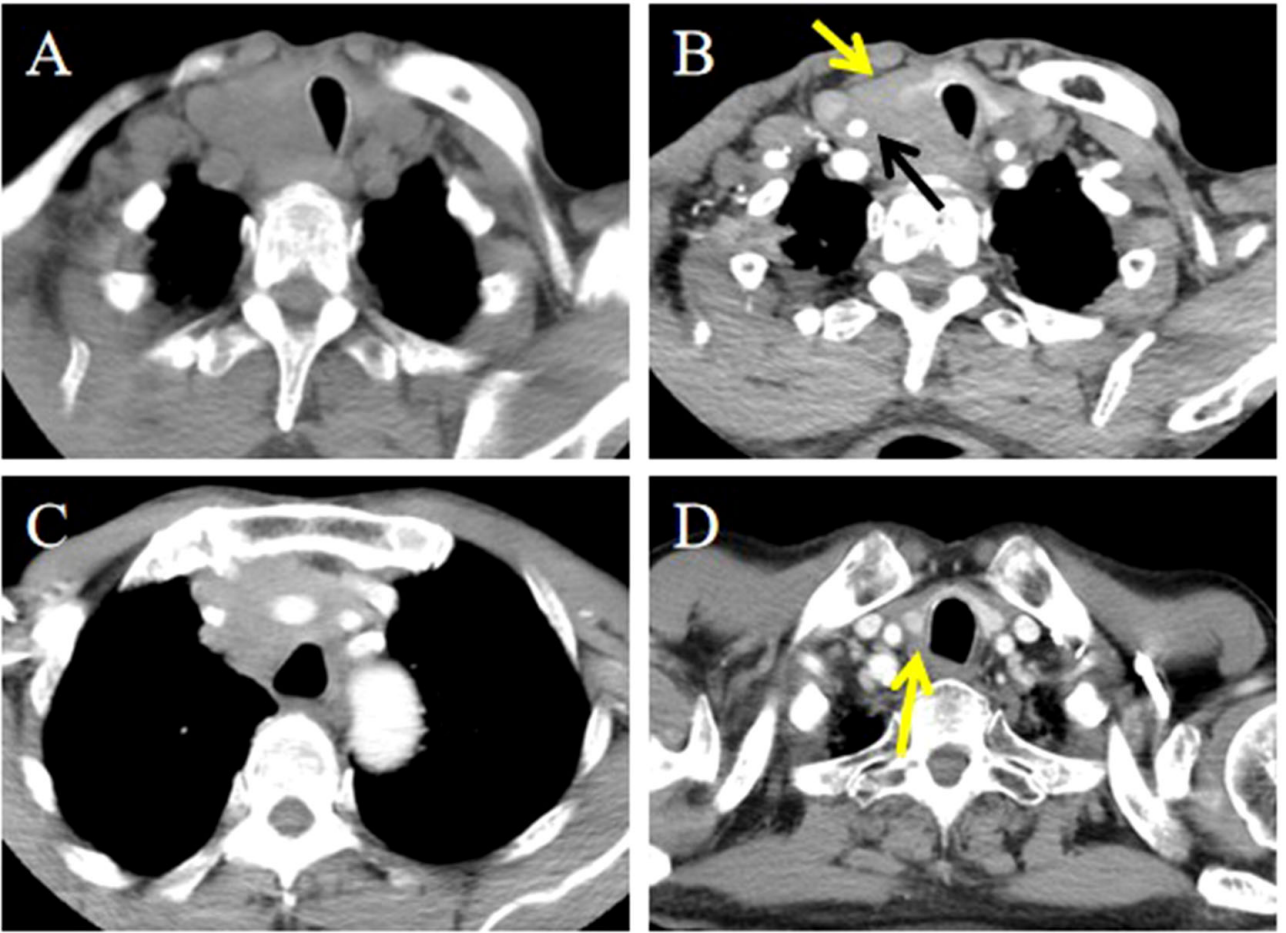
CT imaging of the ITTC before and after treatment. (A) Mass exhibited low soft tissue density on plain CT scans with uniform attenuation; (B, C) Mass demonstrated perfusion-like growth filling the vascular space, infringed strap muscle (yellow arrow); and encased the right common carotid artery (black arrow); (D) After completion of radiotherapy and chemotherapy, imaging modality demonstrated complete remission (yellow arrow).
